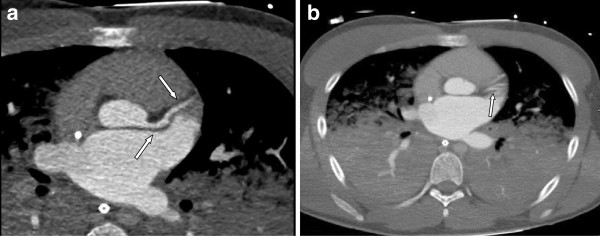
Segment V, VI (a) and IX (b) are shown by computed tomography. The outlet of the LCX is not presentable. Lower lobes are atelectatic and signs of pulmonary edema are visible.
